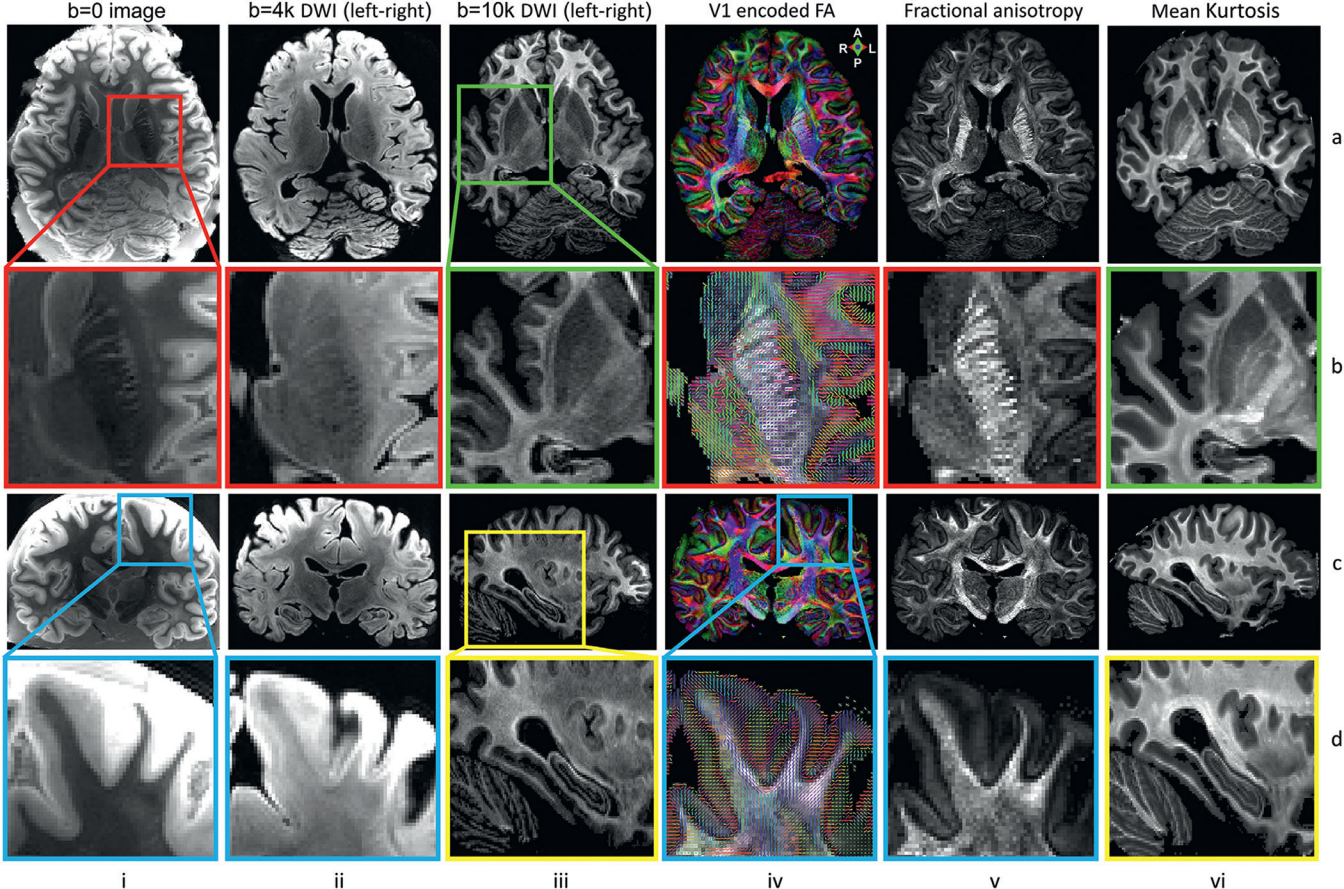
High-resolution DTI and DKI results at 0.73 mm isotropic resolution with *b* = 0 images (column i), diffusion-weighted images (DWI) acquired at *b* = 4000 s/mm^2^ along left-right diffusion-encoding direction (column ii), diffusion-weighted images (DWI) acquired at *b* = 10000 s/mm^2^ along left-right diffusion-encoding direction (column iii), fractional anisotropy (FA) maps color encoded by the primary eigenvectors (V1) from DTI (column iv), FA maps (column v) and mean kurtosis maps (column vi). Row (a) shows axial views of each map. Row (b) shows enlarged regions of interest in the internal capsule (red box) and basal ganglia (green box). Row (c) shows coronal or sagittal views for each map. Row (d) shows enlarged regions of interest in subcortical white matter (blue box) and hippocampus (yellow box). The images highlight the fine-scale gray and white matter anatomy captured by the data, both in deep and superficial brain areas.
